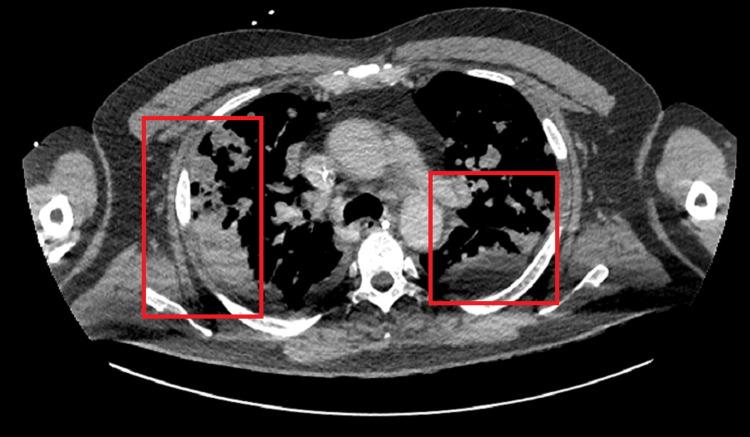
CT scan showing progression of bilateral necrotizing pneumonia in the middle lung zones, with increasing left-sided pleural effusion
